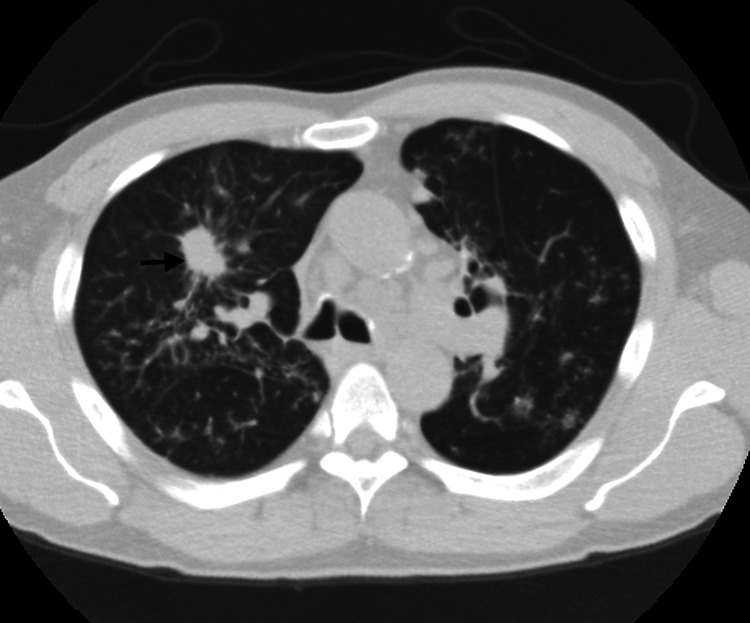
Axial CT chest showing right upper lobe nodule (black arrow)
